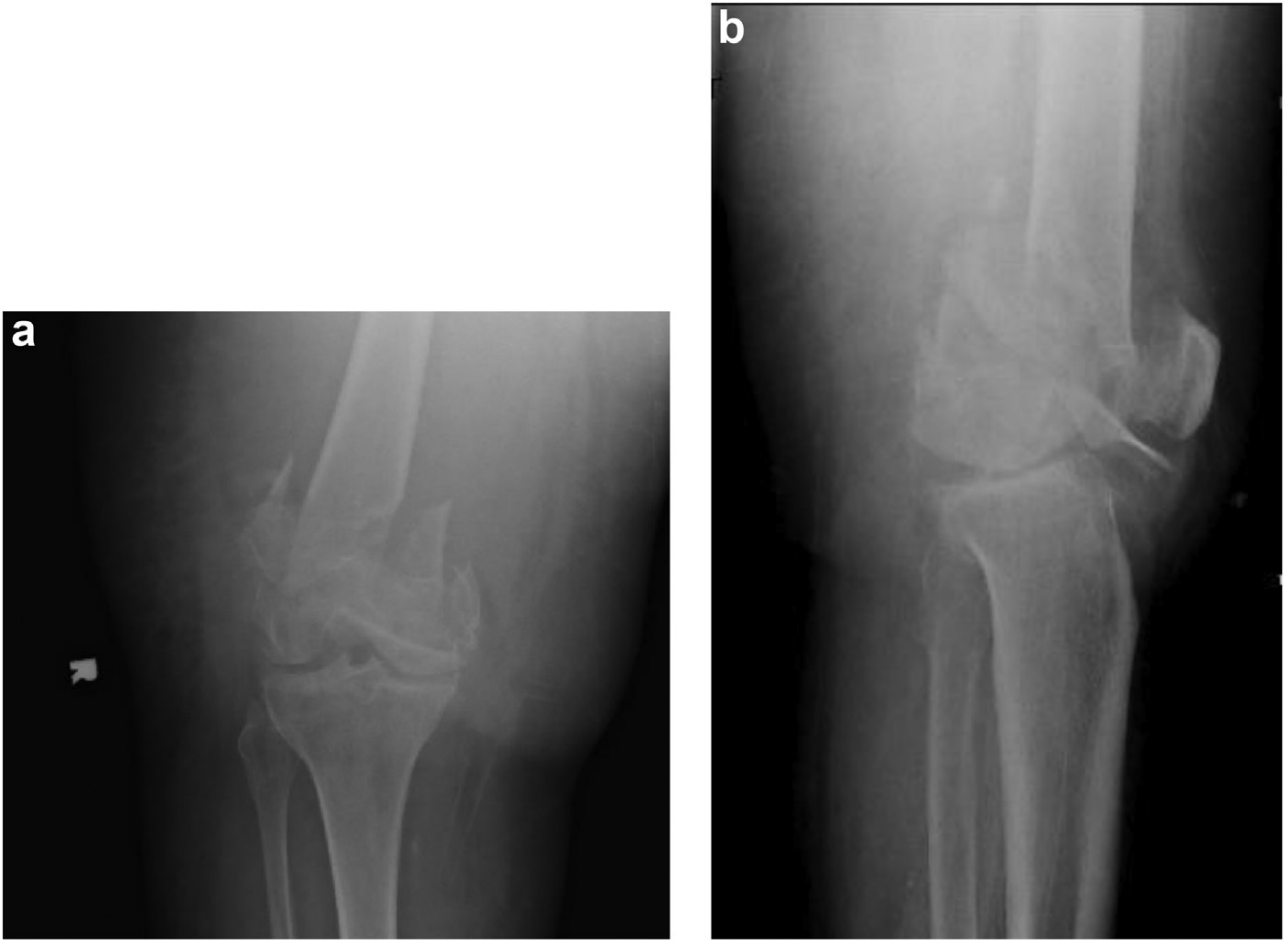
Anteroposterior (a) and lateral (b) injury radiographs demonstrating supracondylar intercondylar distal femur fracture with intercondylar extension with significant comminution of the articular and metaphyseal segments. Insall-Salvati ratio of 1.5.
